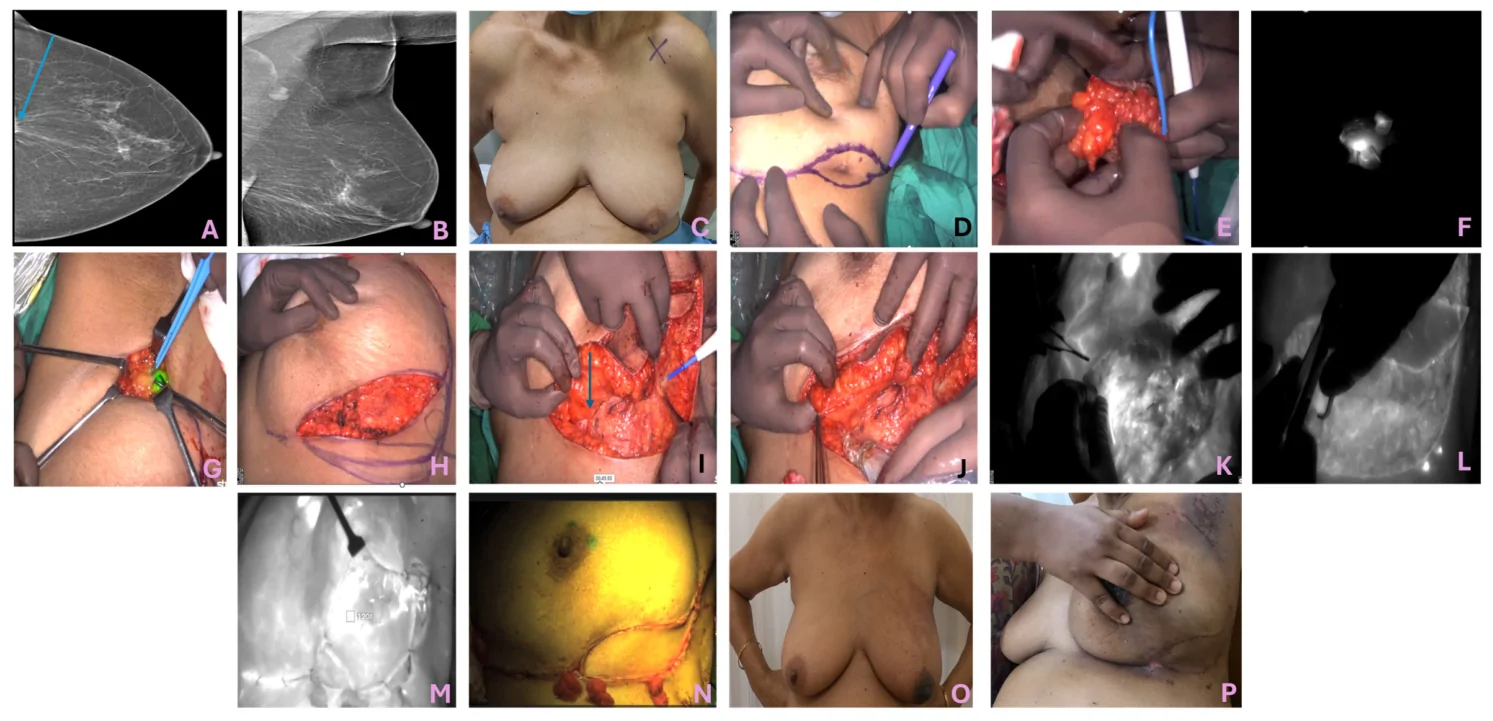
Case 3: Level 3—(A) location of tumor CC image; (B) MLO view; (C) preop image; (D) marking of tumor area; (E) tumor excised; (F) sentinel node detected through ICG dye; (G) sentinel node excised; (H) flap marking done; (I) perforator vessel located; (J) pulsation checked through Doppler; (K) vascularity of the flap checked; (L) vessel clamped; (M) perfusion score of the flap assessed; (N) flap closure, wound closed; (O,P) postop images.
